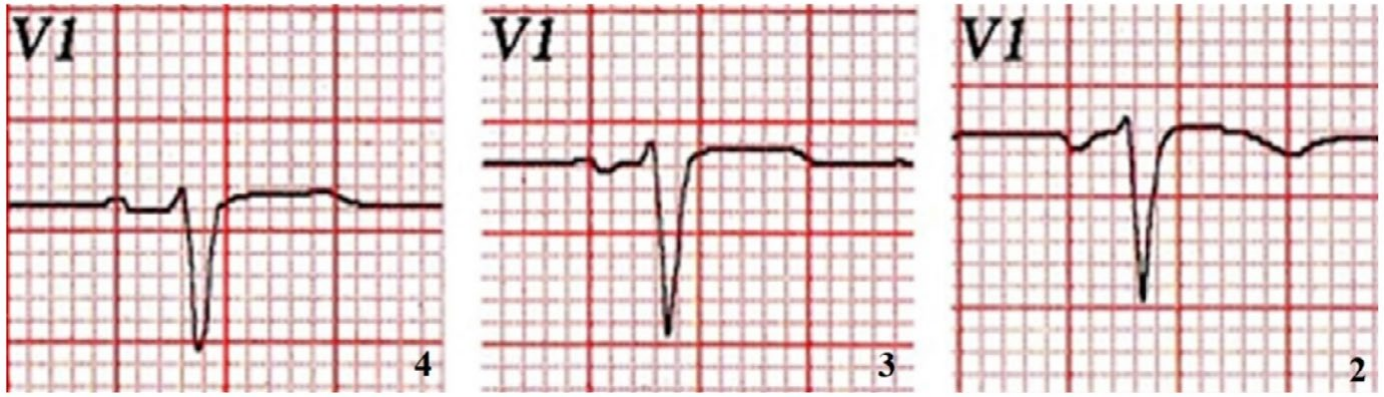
V1 lead of a healthy patient with normal left atrial size for echocardiography, with the V1 electrode located in the correct place: fourth intercostal space (4), third intercostal space (3), and second intercostal space (2). Note how a normal P wave becomes progressively negative as the V1 electrode is located upward
